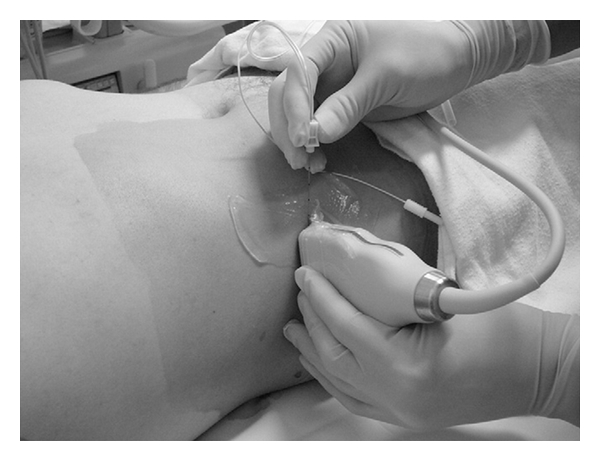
Ultrasound-guided TAP block showing needle alignment and ultrasound transducer placement on skin using an in-plane technique [20]. Reproduced with permission.
